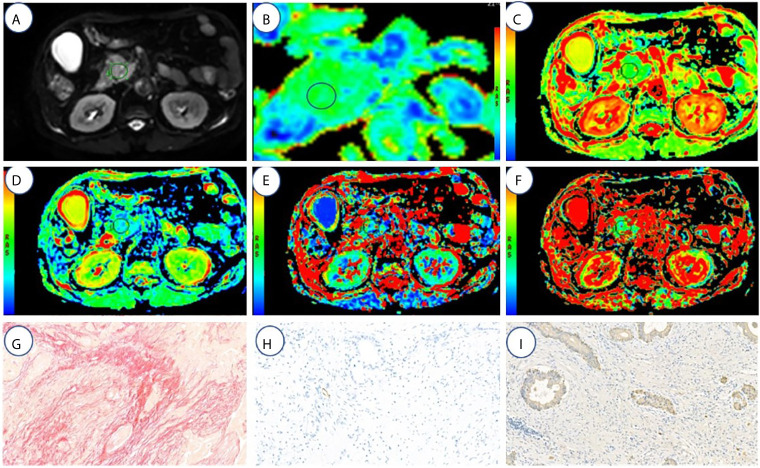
A 67-year-old male with high fibrosis PDAC. (A–F) represents the DWI, ADC(0,500), ADC(0,800), D, f, and D* values map, respectively. (G–I) represents the fibrosis, microvascular density, and tumor cell dyeing map, respectively. ROI was set in PDAC on DWI, ADC _(0, 500)_, ADC _(0, 800)_, D, f, and D* value maps. The cancer tissues show different signals from normal tissues in the DWI, ADC _(0,500)_ (1.3 μm^2^/ms), ADC _(0,800)_ (1.2 μm^2^/ms), D (1.16 μm^2^/ms), f (18.03%), and D* (64.5 μm^2^/ms). Fibrosis, MVD, and tumor cell are 47, 1.3, and 21%, respectively.
